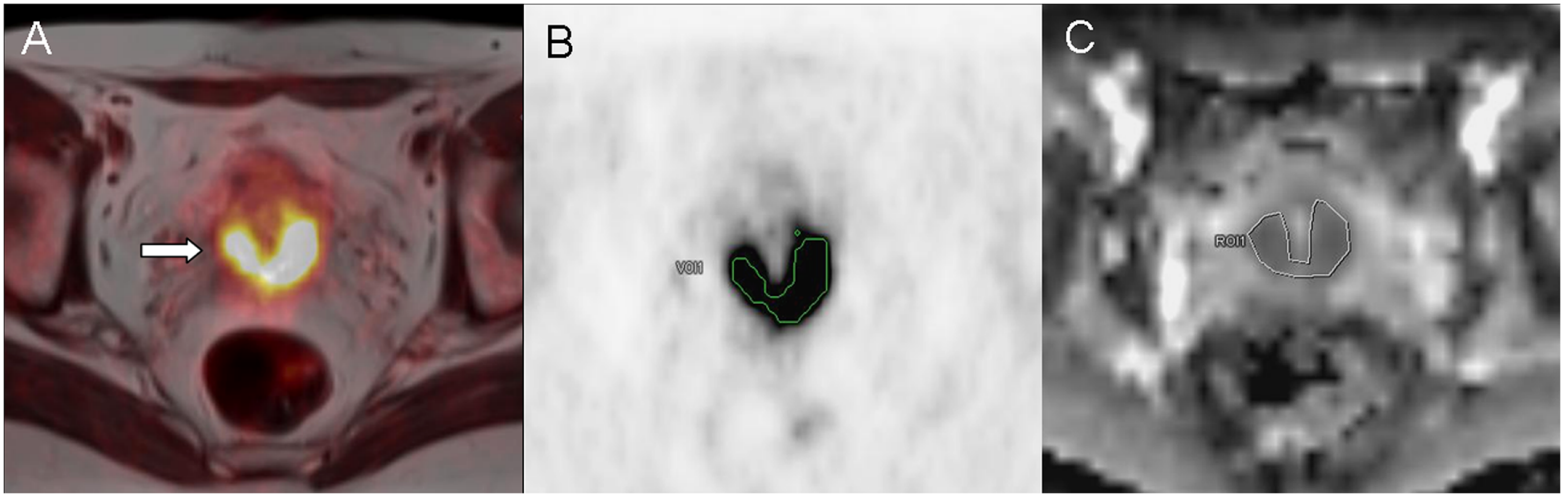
Lesion detection and definition of tumor margins (arrow) of primary cervical cancer in fused PET/MRI T2 –HASTE–sequence (A). Manual placement of a polygonal VOI in attenuation corrected PET images (B). ROI adjustment by manual segmentation on (C) the corresponding MRI ADC—map.
